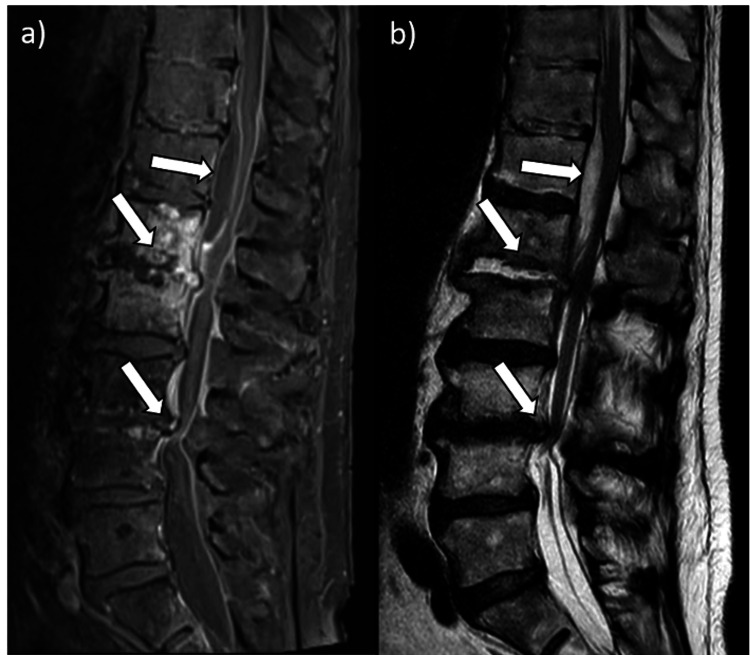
Lumbar MRI at presentation a) Sagittal T1-weighted sequence with contrast and b) Sagittal T2-weighted sequence revealing a spinal epidural abscess (SEA) at level Th12/L1, spondylodiscitis at level L1/2 and stenosis of the spinal canal at L3/4
